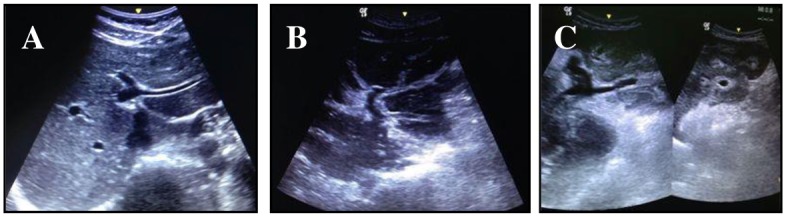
Abdominal ultrasound images of periportal fibrosis patterns. (A) Peripheral periportal thickening + central periportal thickening. (B) Advanced peripheral periportal thickening + central periportal thickening. (C) Very advanced central and peripheral periportal thickening.
